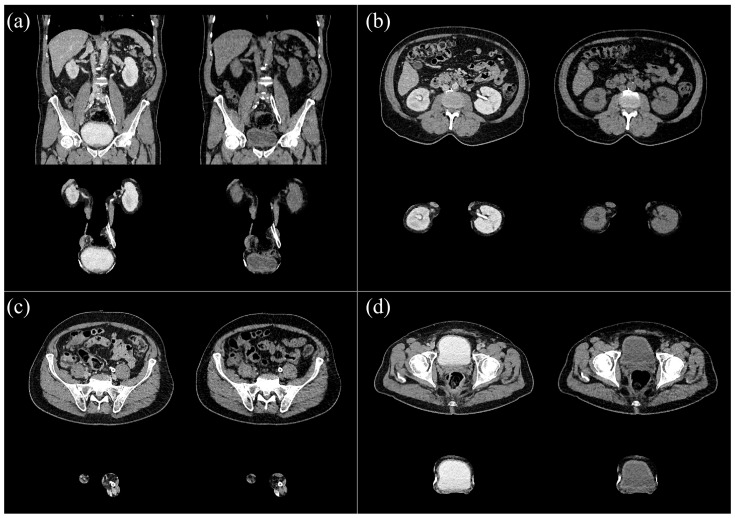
Example of the performance of focused view CTU method 3 (expanded boundary and extrapolation method) on coronal slices (a) and several axial slices (b–d), with contrast-enhanced and unenhanced CT images shown in each panel.
